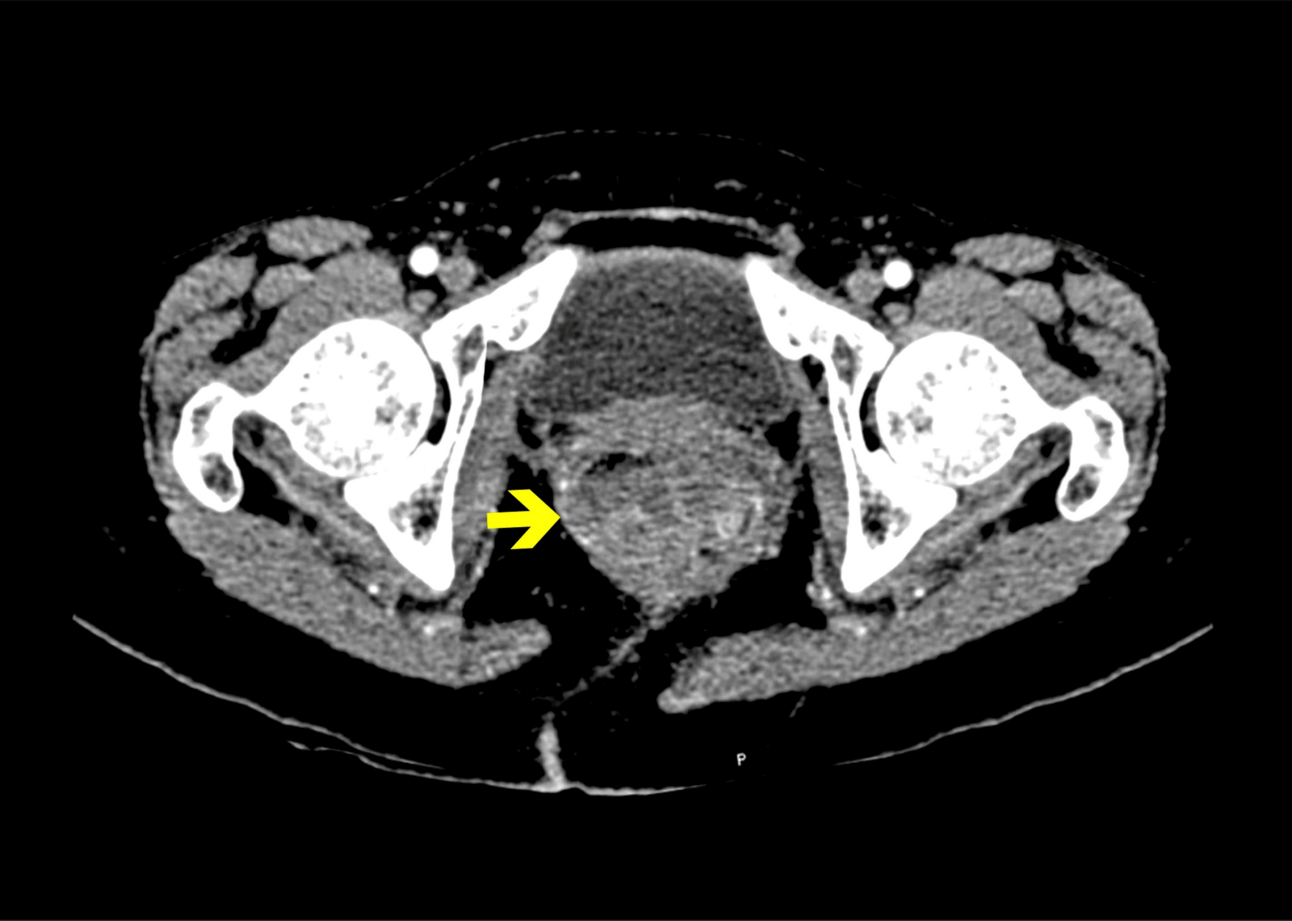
Axial CT image (contrast-enhanced). Axial contrast-enhanced computed tomography (CT) of the pelvis showing a soft tissue mass occupying the rectal lumen, with irregular borders and apparent infiltration into the mesorectal fat. The lesion appears hypodense compared to the surrounding muscle tissue. There is no clear evidence of pelvic bone erosion. Bilateral femoral heads and acetabula are intact.
